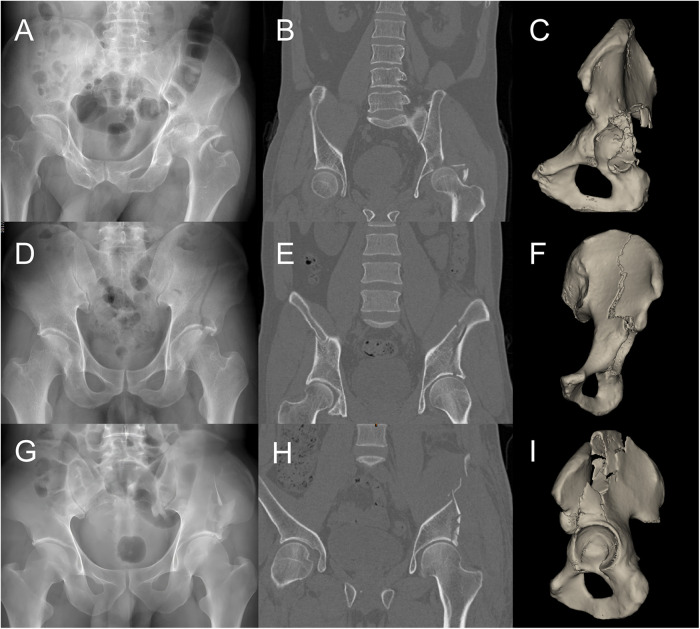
Representative radiographic, CT, and three-dimensional images of A3 acetabular roof injuries. (A–C) A3.1 roof wall fracture. (D–F) A3.2 roof column fracture. (G–I) A3.3 complex roof column fracture. For each subtype, the panels show an anteroposterior pelvic radiograph, a coronal CT image, and a three-dimensional reconstruction from one representative patient. Plain radiographs show suspected acetabular roof involvement, whereas coronal CT and three-dimensional reconstruction clarify the subtype-specific fracture morphology.
